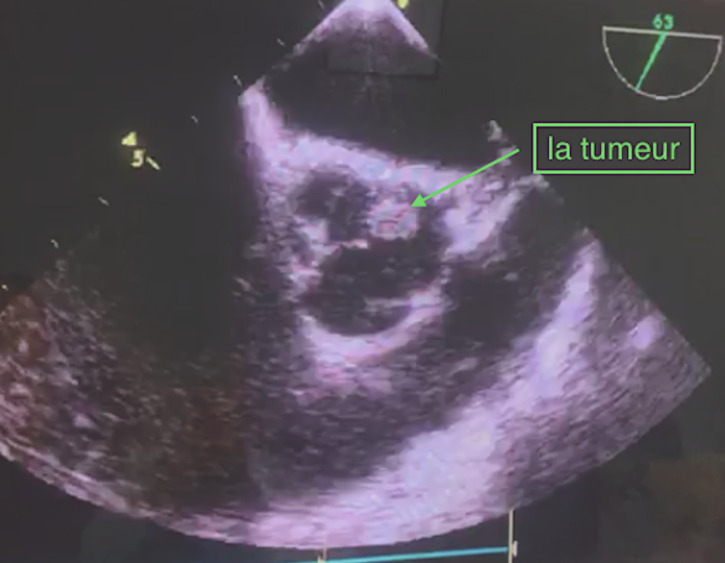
transoesophageal echocardiography short axis view, arrow points to a 10mm /8mm tumour, attached to the non/left commissure
